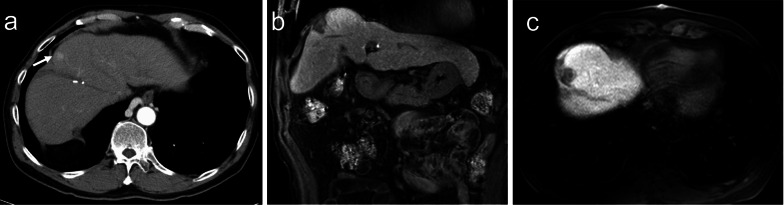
a CT image of hepatocellular carcinoma at medical segment. It was well enhanced in the arterial phase (arrow). b MRI image of post-RFA for hepatocellular carcinoma at medical segment (coronal view). c MRI image of post-RFA for hepatocellular carcinoma at medical segment (axial view)
